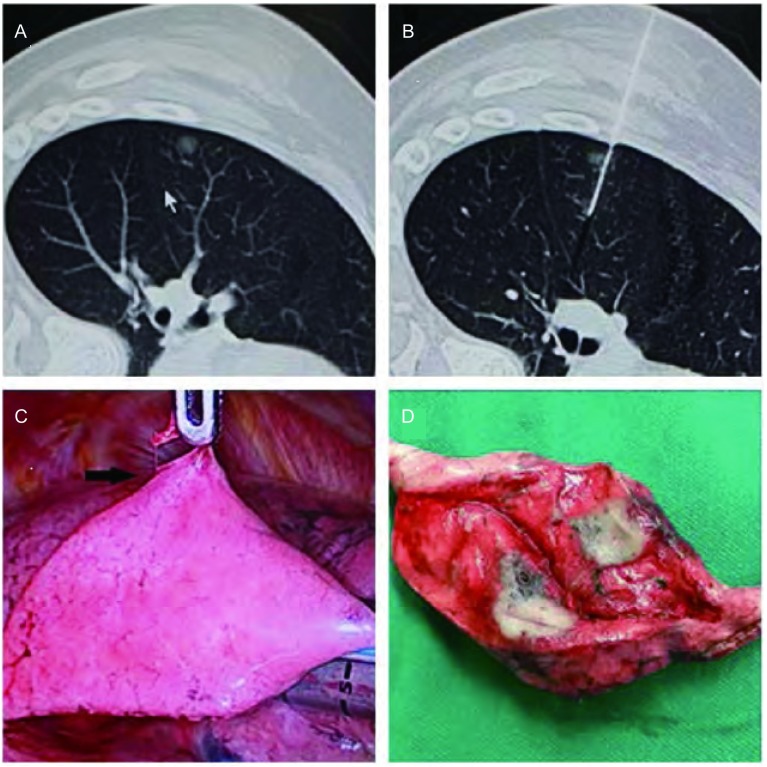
GGO病灶的CT定位扫描图及VATS切除。A：肺GGO病灶的CT扫描图；B：穿刺针定位于肺GGO病灶的CT扫描图；C：Hook-wire定位针引导肺GGO病灶的VATS切除；D：切除的肺组织中见直径7 mm病灶。 CT scanograms and VATS resection of the GGO lesionswith pilot pin. A: CT scanograms of the GGO lesions; B: CT scanograms of the GGO lesions with pilot pin; C: VATS resection of GGO lesions guided by Hook-wire pilot pin; D: Lesions (d=7 mm). VATS: video-assisted thoracic surgery; CT: computed tomography.
